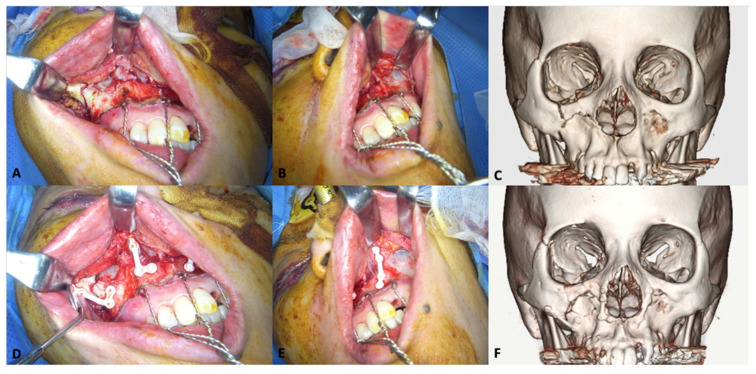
Representative clinical photographs of the u-HA/PLLA fixation system in the midfacial region. A 55-year-old woman exhibited complex maxillofacial fractures, including Le Fort I maxillary fracture, zygomaticomaxillary complex fracture, and orbital floor fracture on the right side, and maxillary sinus fracture on the left side. The Le Fort I maxillary fractures were fixed using u-HA/PLLA plates. (A), Complex fractures on the right side of the maxilla. (B), Maxillary fracture on the left side. (C), Preoperative CT scan image. (D), Two L-shaped u-HA/PLLA plates were used to fix fractures on the left side. (E), A u-HA/PLLA straight plate was implanted to correct the fracture on the left side. (F), Six-month postoperative CT scan image.
